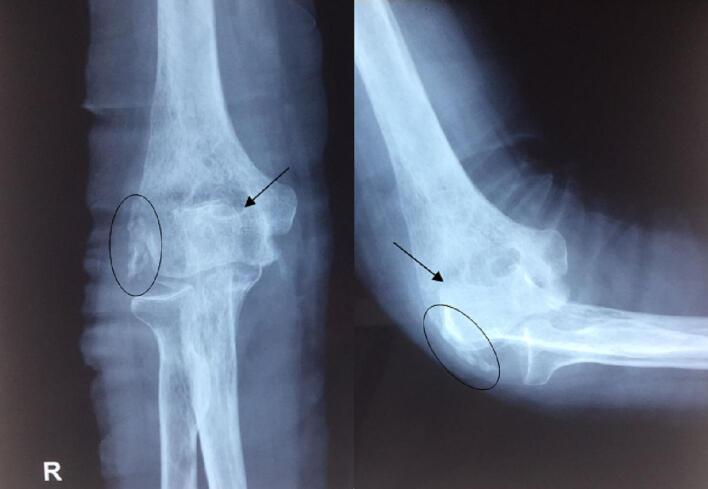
Right anteroposterior and lateral elbow X-ray. Image showing neglected dislocation of the elbow joint (arrow) with soft tissue calcification (circle).
